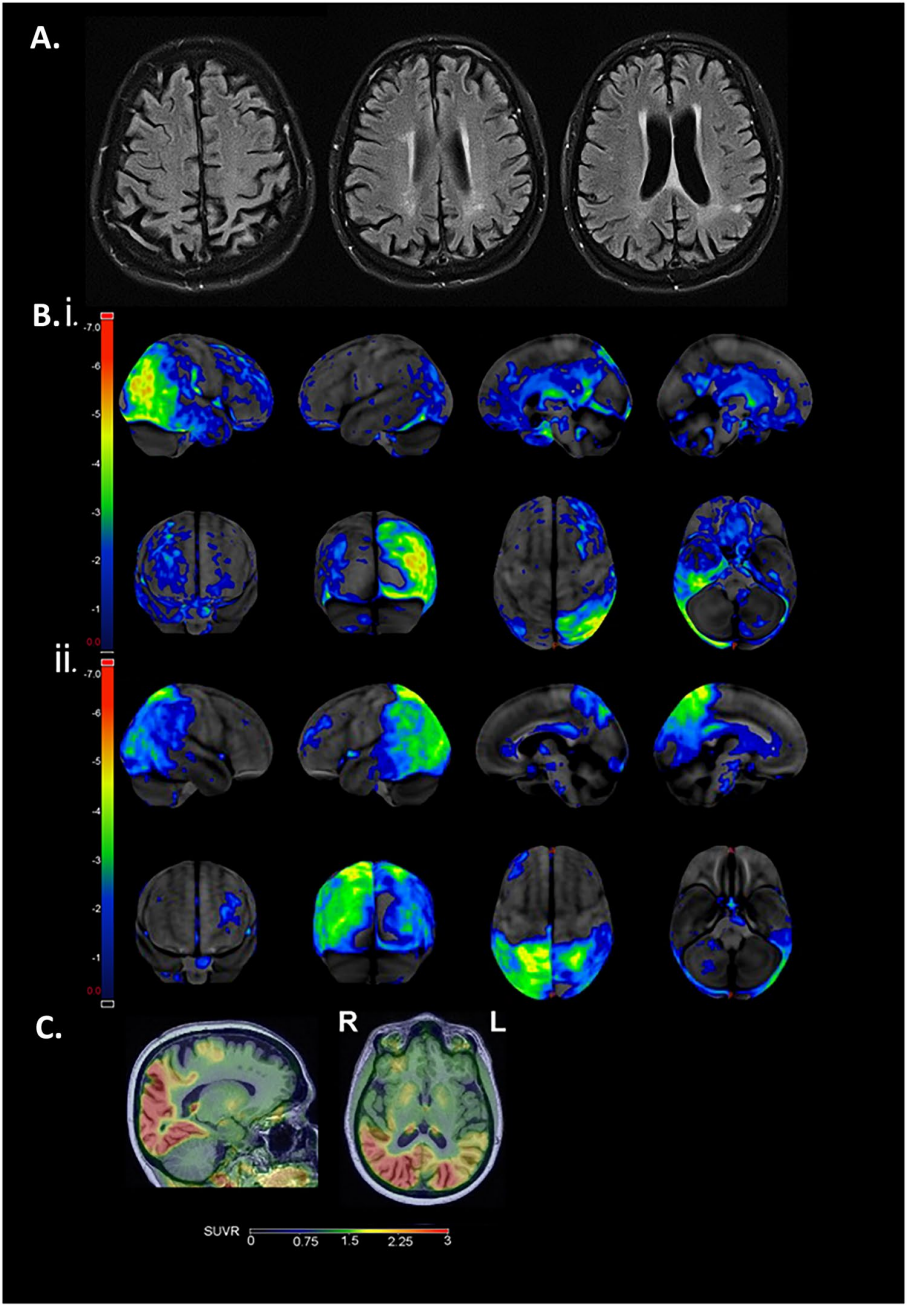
A T2 axial FLAIR MRI demonstrating parieto-occipital atrophy in a patient with PCA. B Fluorodeoxyglucose (FDG)-positron emission tomography (PET) scans with statistical maps showing regions of significant hypometabolism relative to age-matched controls (GE cortex ID): i PCA patient with predominantly right occipital-parietal-temporal hypometabolism with prominent environmental agnosia and dressing apraxia and ii PCA patient with predominantly left occipital-parietal-temporal hypometabolism with prominent Gerstmann features including acalculia. C Tau-PET (flortaucipir) scan with predominant parieto-occipital tracer uptake in a patient with PCA.
